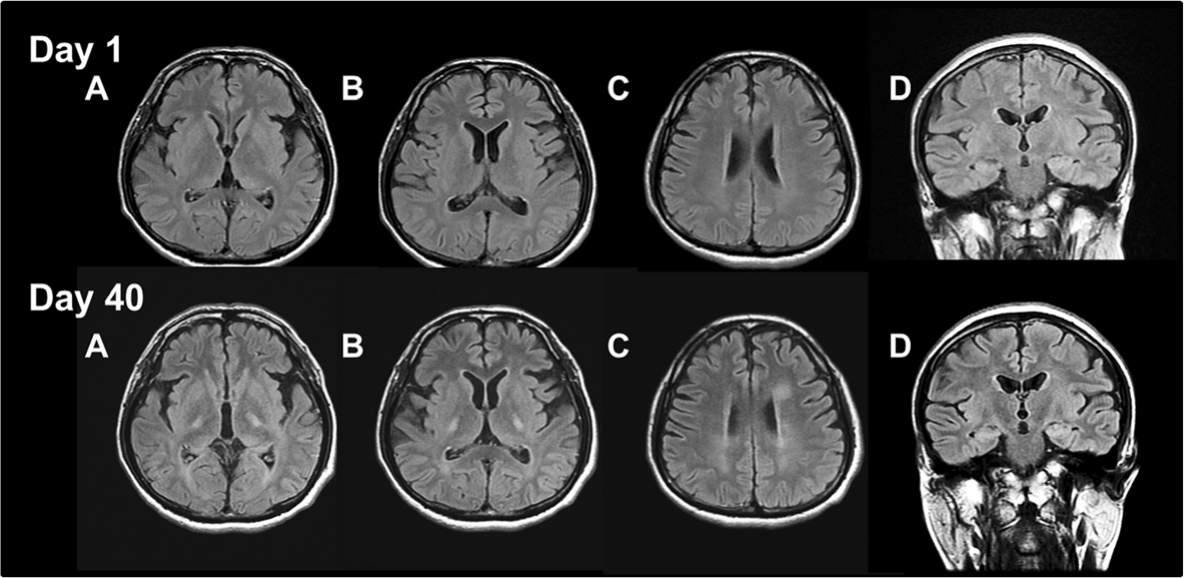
FLAIR MR images of the brain on day 1 and day 40 after admission. The images on day 40 show that hyperintense foci are unequivocally present in the bilateral internal capsules (Day 40, a, b and d), periependymal regions of the posterior horns of lateral ventricles (Day 40, a, b and d), and cerebral white matter near the lateral ventricles (Day 40, c)
